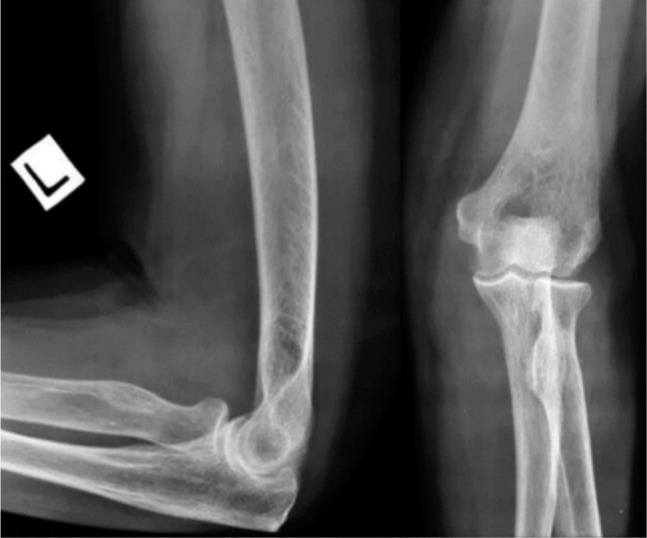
Radiographs of elbow (Lateral and AP) 3 months post trauma showing concentric left elbow joint.
